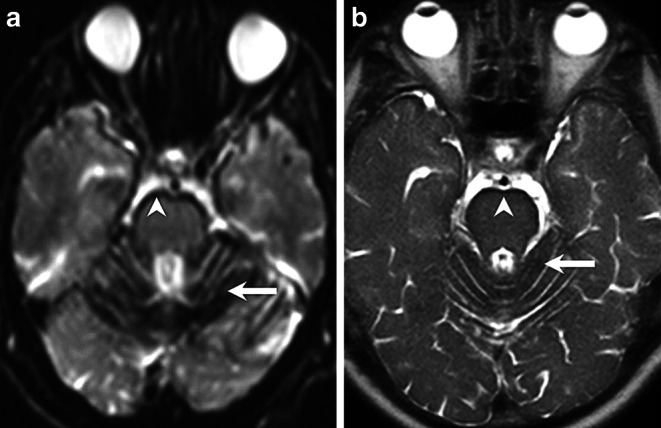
Axial diffusion-weighted (b1000, a) and axial T2-weighted images (b) show extensive superficial siderosis along the brainstem (*arrowhead*) and the cerebellar folia (*arrow*)
